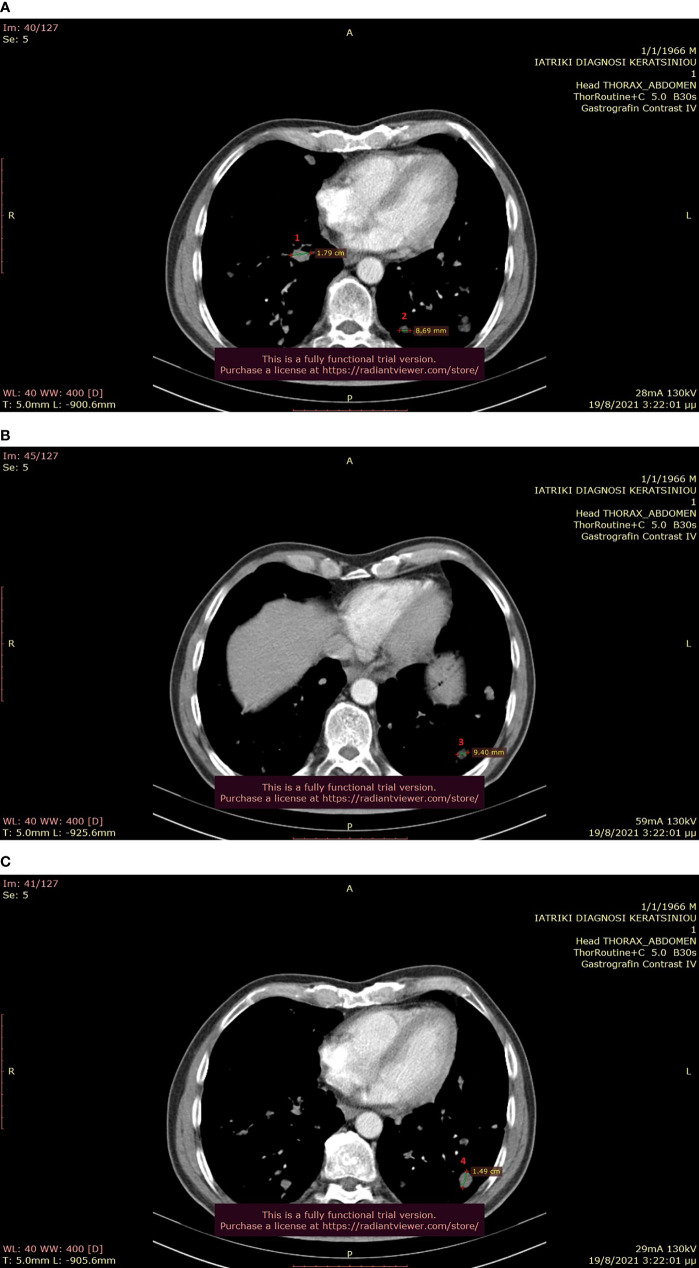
(A–C): Thoracic CT after the completion of 6 treatment courses, showing SD in the lung (August 2021).
